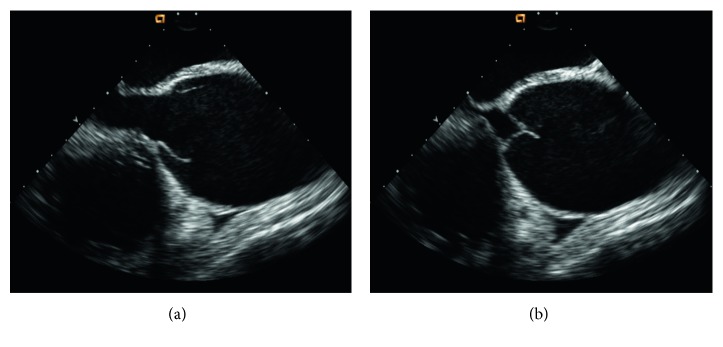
Transesophageal echocardiography (midesophageal, long-axis view) showing massive dilation of ascending aorta. (a) Opening of the aortic valve; (b) closure of the aortic valve.
